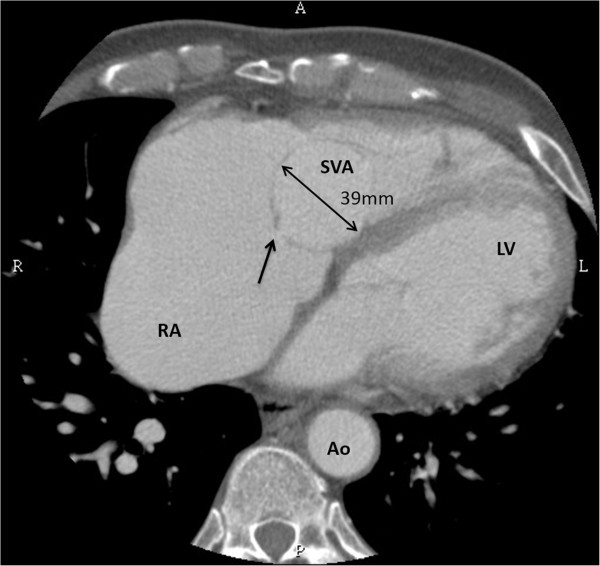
Computed tomography showed a defect (*arrow*) from aneurysm (An) to right atrium (RA). LV indicates left ventricle; Ao, aorta.
